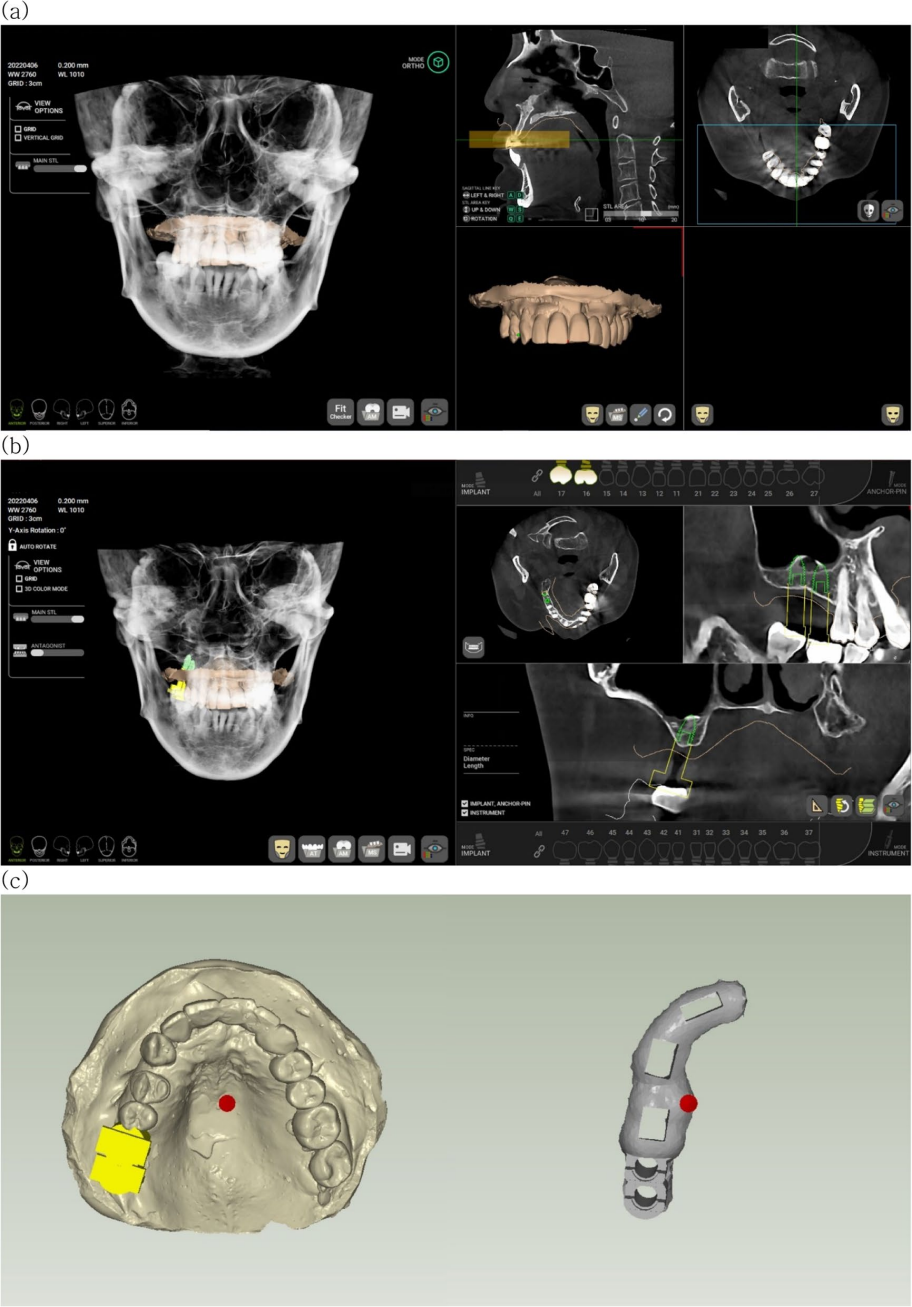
Protocol for implant surgical guide preparation using R2GATE^®^ software. a CBCT and STL matching. b Implant planning. c Guide design
